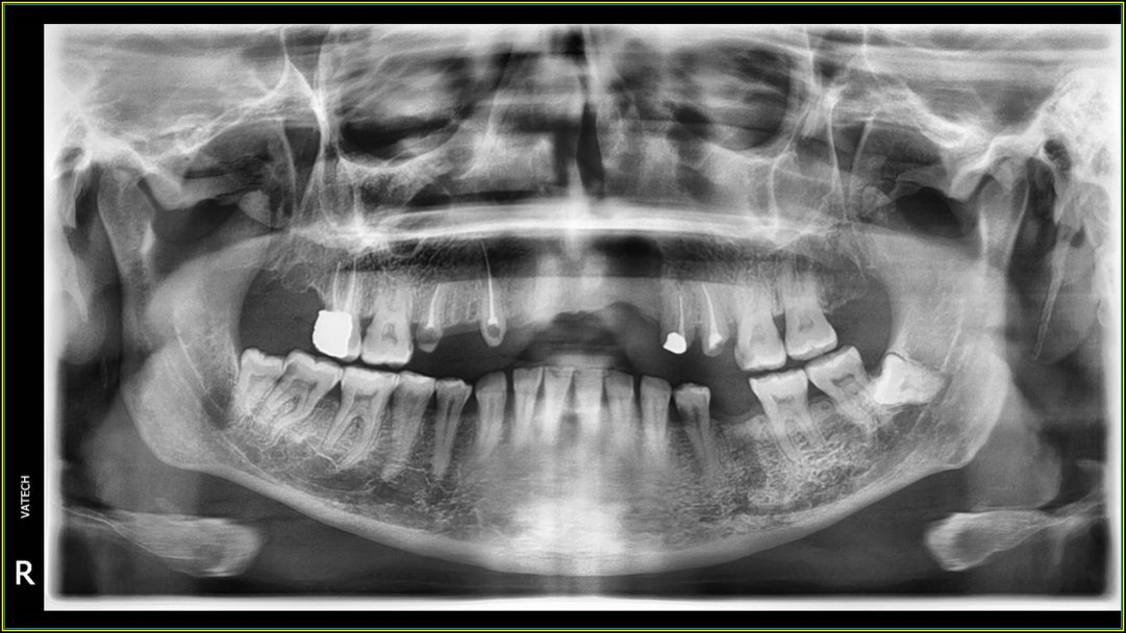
Postoperative radiography.
